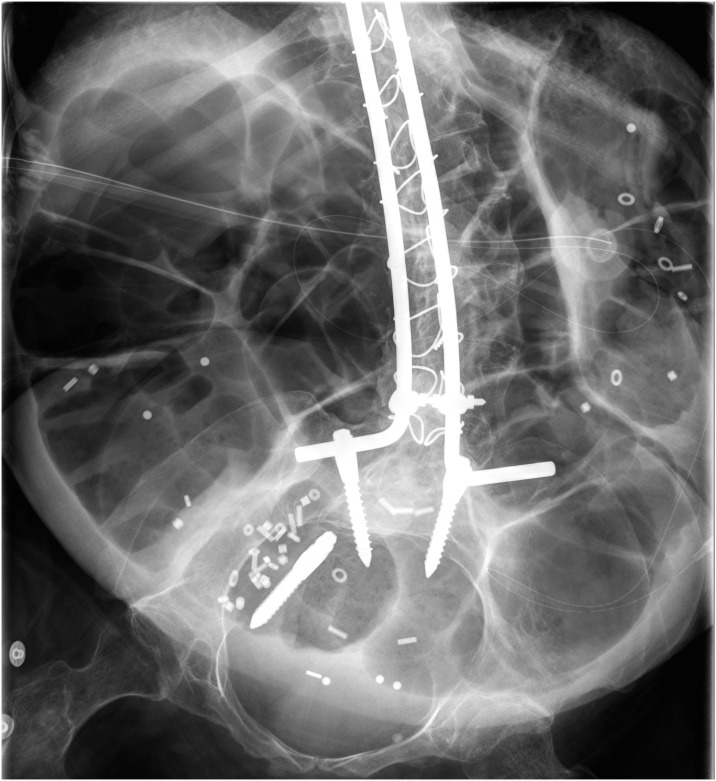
Representative radiograph of the abdomen. This is a representative radiograph of a not acutely ill, 38 year old patient with Duchenne muscular dystrophy. Radiopaque markers and extensive meteorism are seen in the colon. Rods and screws (one of them displaced) for spinal stabilization and a PEG tube are visible.
